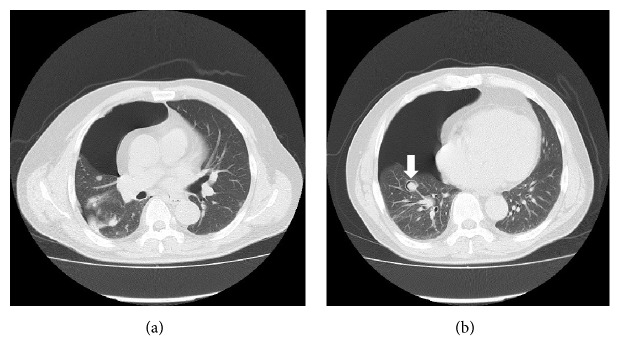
(a, b) Follow-up computed tomography on day 34 of lenvatinib start showed the right pneumothorax and the air was recognized inside a part of lung metastases (arrow).
